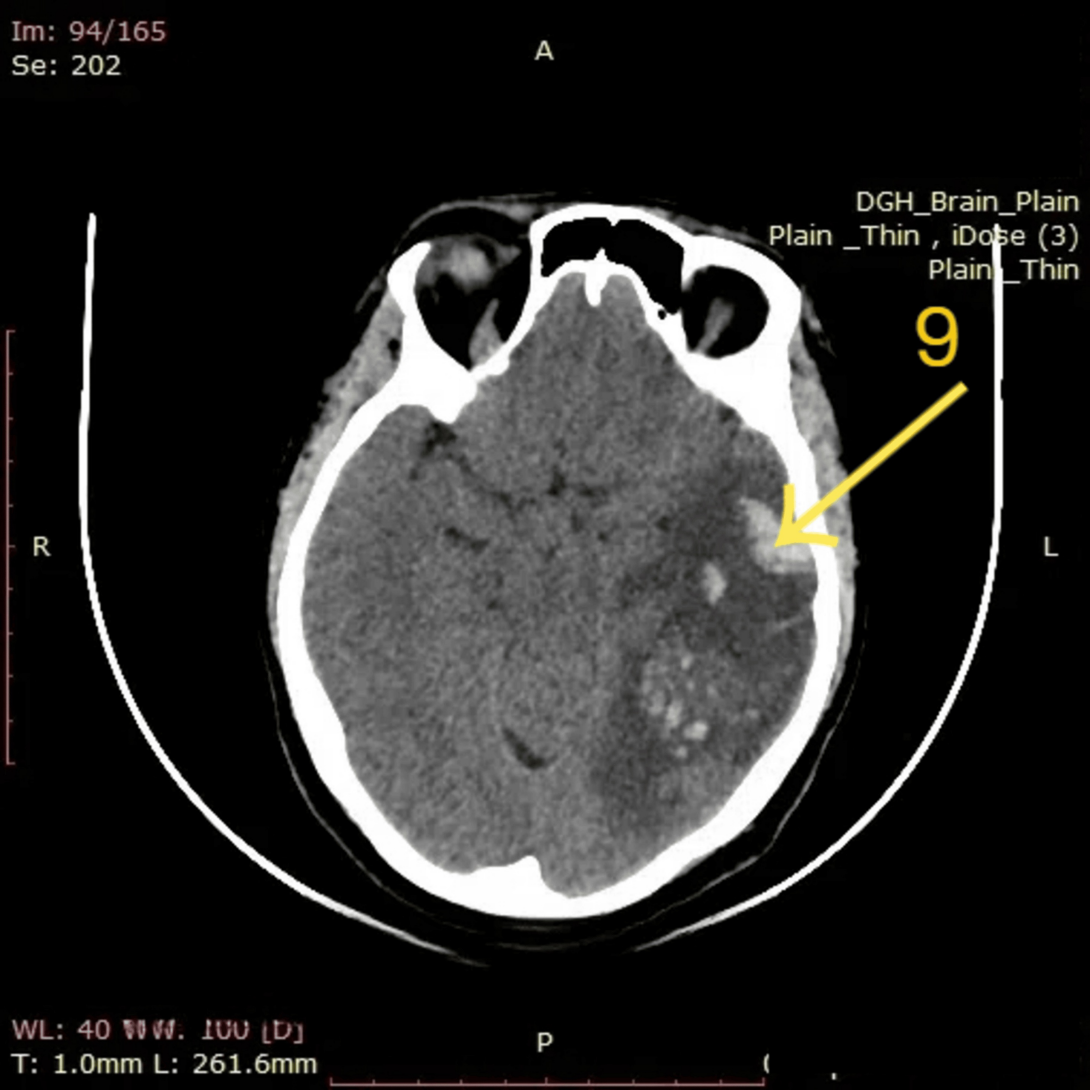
Non-contrast CT (NCCT) brain two days after starting anticoagulation Arrow 9: Well-defined hyperdense area in the left temporal lobe. This hyperdensity is consistent with resolving hemorrhagic venous infarction in the context of cerebral venous sinus thrombosis (CVST).
